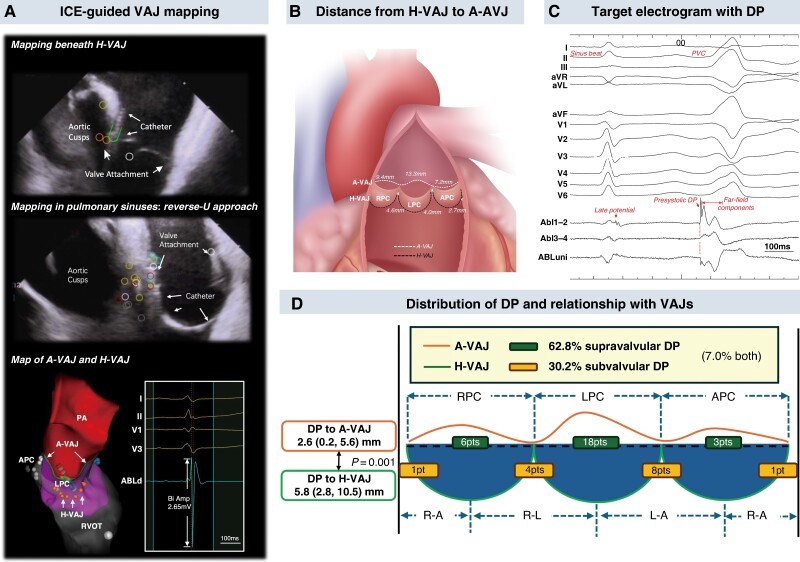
Intracardiac echocardiography-assisted electroanatomical mapping for ventricular arrhythmias from RVOT. (*A*) Catheter position (conventional and reverse-U approach) during ICE-guided VAJ mapping at different locations and the demonstration of H-VAJ and A-VAJ on the 3D map. (*B*) Distance from H-VAJ to A-VAJ at different locations. (*C*) Typical target electrogram with DP in RVOT-PVC. (*D*) Distribution of DPs and their relationship to H-VAJ and A-VAJ. APC, anterior pulmonary cusp; A-VAJ, anatomic ventriculo-arterial junction; DP, discrete potential; H-VAJ, haemodynamic ventriculo-arterial junction; ICE, intracardiac echocardiography; LPC, left pulmonary cusp; PA, pulmonary artery; PVC, premature ventricular contraction; RPC, right pulmonary cusp; RVOT, right ventricular outflow tract.
